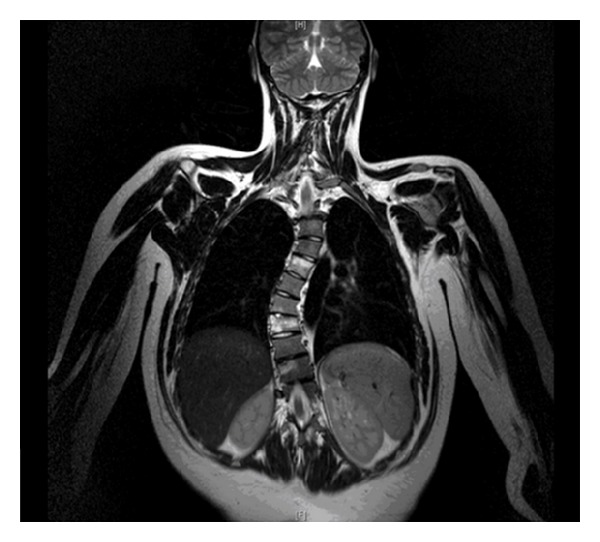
Coronal Thoracic Spine MRI image, T2W. Abnormal high signal in T5 and T8 vertebral bodies with right atypical thoracic scoliosis.
